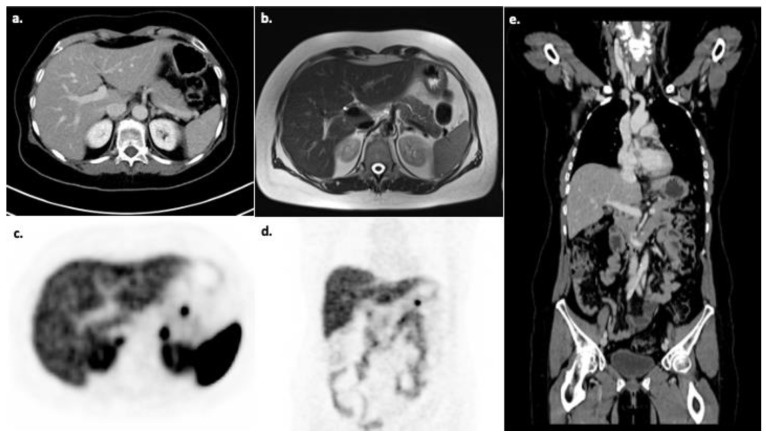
Patient from our institution with clinically suspected neuroendocrine tumor (NET). ^68^Ga-DOTA-TATE PET/CT imaging and MRI were performed for diagnostic work-up. (a) CT in venous phase is unremarkable and shows homogeneous liver parenchyma as well as normal pancreatic parenchyma. (b) T2-weighted MRI of the same patient also shows homogeneous liver parenchyma and normal pancreatic parenchyma with no clear evidence of malignancy. (c) ^68^Ga-DOTA-TATE PET in the axial plane reveals a markedly elevated somatostatin receptors (SSR) expression in line with a small tumor in the pancreatic tail in line with diagnostic PET features of a NET. Physiological SSR expression of the adrenal glands can also be noted. (d) ^68^Ga-DOTA-TATE PET in the coronal plane visualizes the NET tumor clearly and the anatomical position of the tumor in the pancreatic tail. (e) Coronal CT imaging can be used for further planning of potential surgical treatment, although the tumor cannot be visualized solely relying on CT imaging.
